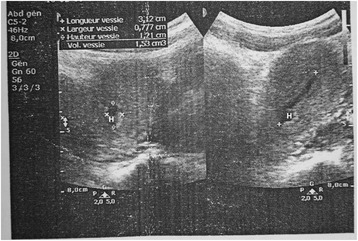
Showing Hydrometra (H) on two-dimensional pelvic ultrasound scan
